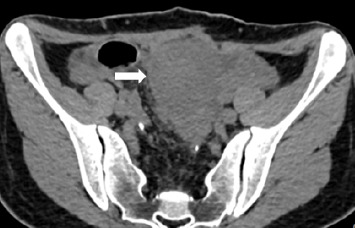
CT-scan showing a large (5.57 × 5.09 cm) intra-abdominal desmoid tumor.
